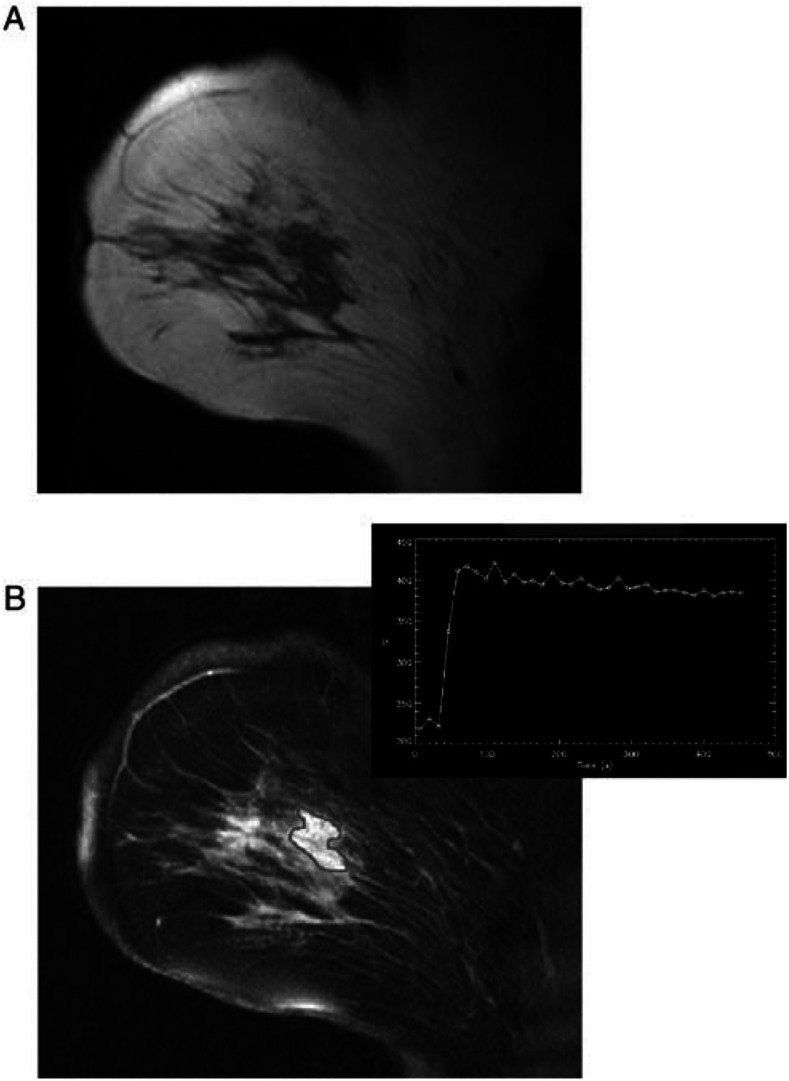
T1-weighted image of DCIS (A) and fat-saturated image after gadolinium contrast (B) with malignant signal-intensity curve of enclosed area.
